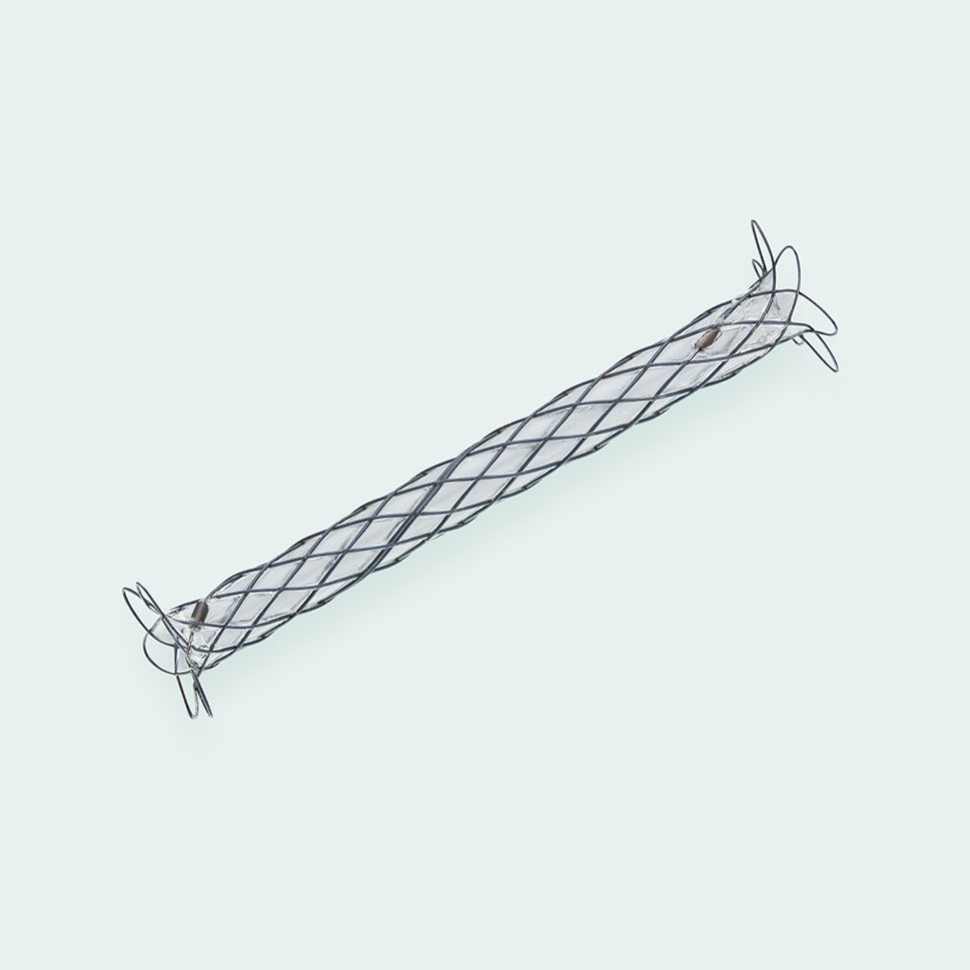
Somatex^®^ Intra Uterine Shunt. The shunt consisting of a nitinol wire mesh and impermeable silicone coating with silicone-free, self-deploying parasols at both ends of the shunt with two X-ray markers
